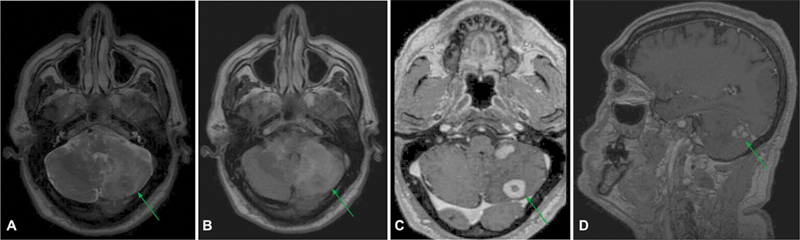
Magnetic resonance imaging scan showing expansive intraparenchymal nodular lesions located in the posterior fossa, involving the pons, peduncles, and cerebellar hemispheres, presenting mild hypointense signal in the T2-weighted sequence (
